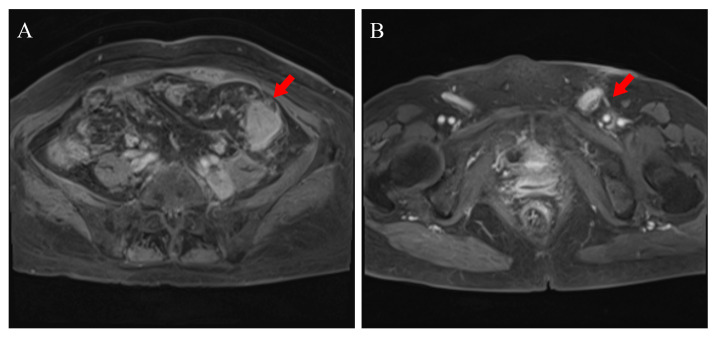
Contrast-enhanced abdominal MRI. (A) Axial fat-suppressed T1-weighted image (portal venous phase) shows irregular circumferential thickening and marked enhancement of the descending colon wall. (B) Axial fat-suppressed T1-weighted image reveals enlarged, heterogeneously enhancing lymph node clusters in the left inguinal region.
